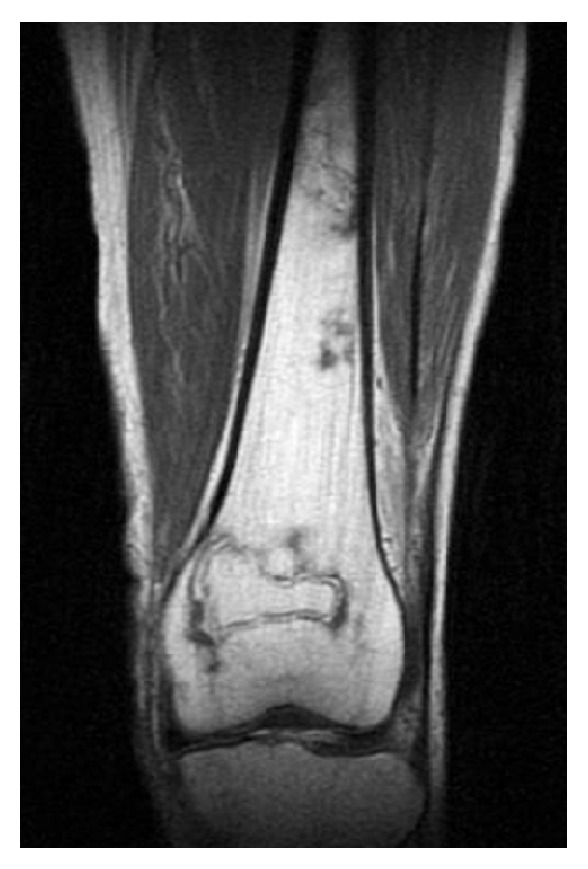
T1W coronal section MRI showing multiple demarcated bone marrow abnormalities with double-line sign, compatible with osteonecrosis.
